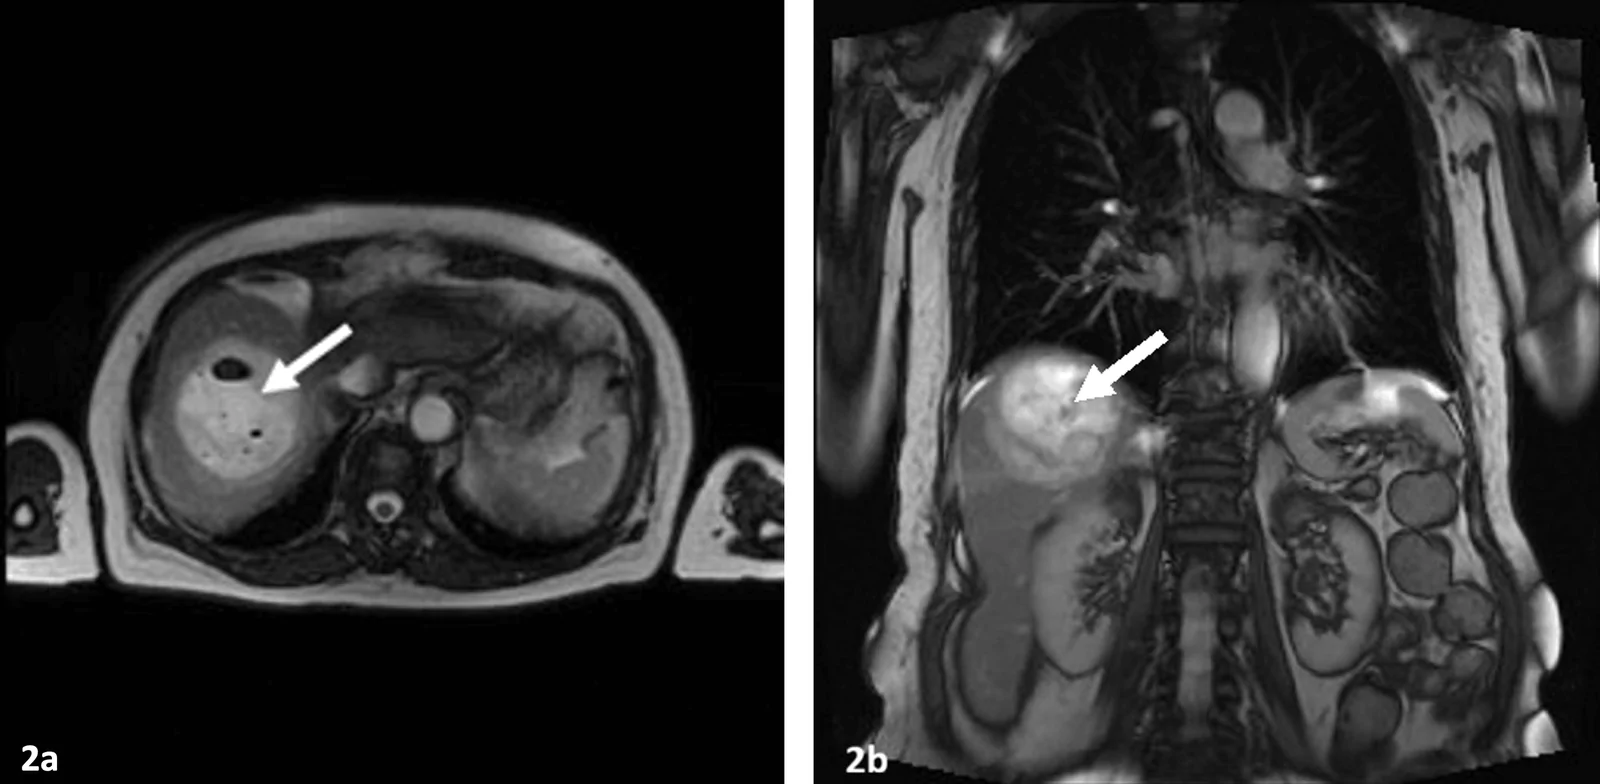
T2-weighted magnetic resonance images of the right hepatic lobe lesion The arrows indicate a heterogeneous lesion with central hyperintensity corresponding to extensive central necrosis in the clinical context of metastatic melanoma.
